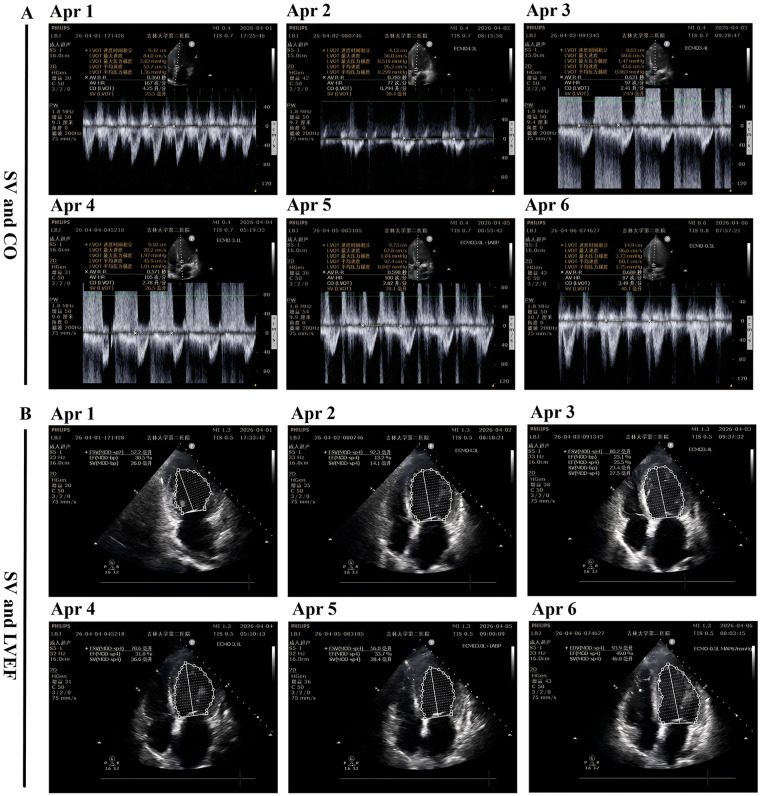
Dynamic bedside echocardiographic assessment of CO and LVEF during ECMO support. (A) From April 1 to April 6, the velocity-time integral was measured using pulsed-wave Doppler of the left ventricular outflow tract, and SV and CO were calculated. (B) From April 1 to April 6, left ventricular volumes were measured using the single-plane modified Simpson method in the apical four-chamber view, and SV and LVEF were calculated.
